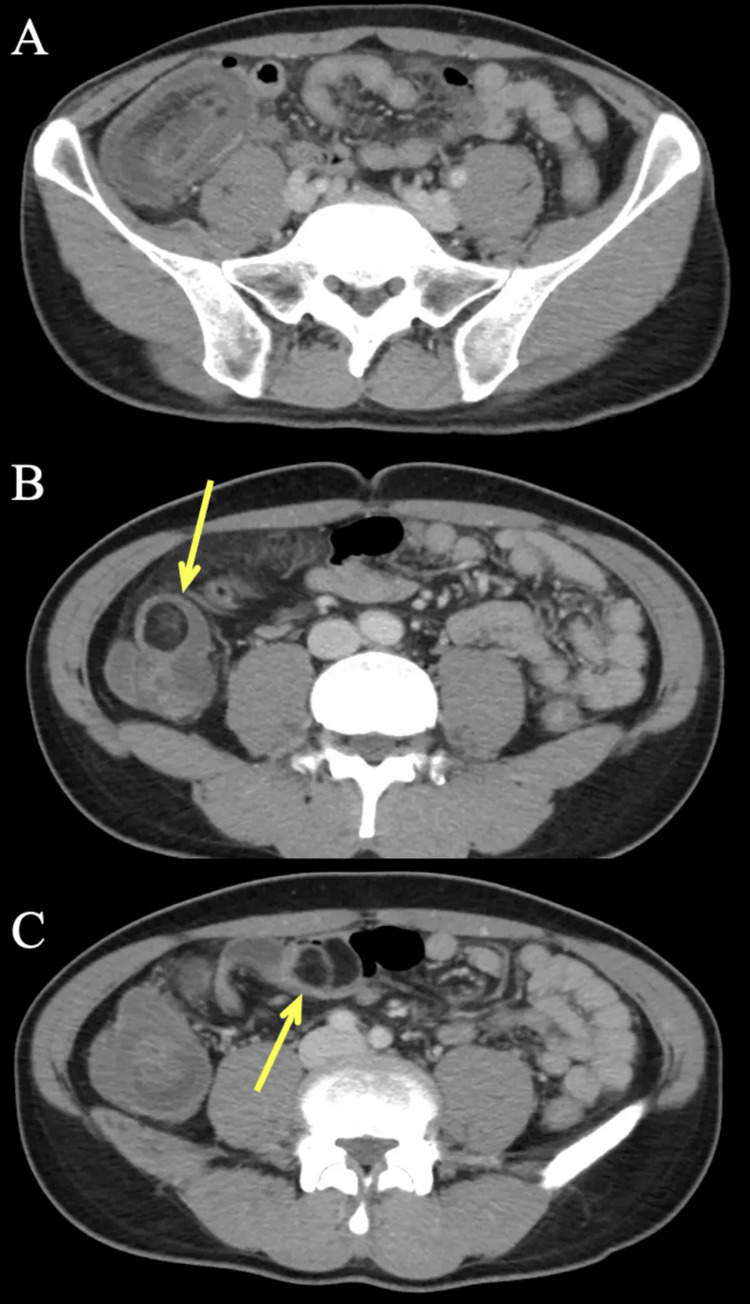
Ileocolic intussusception with terminal ileum lipomas Axial CT abdomen and pelvis slices showing the ileocolic intussusception (A) with terminal ileum lipomas (yellow arrows) both within (B) and outside (C) the intussusception.
